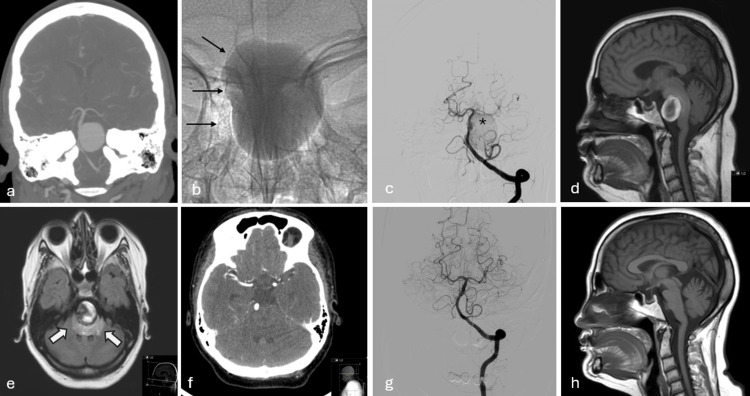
a: Coronal reformatted computed tomography angiogram shows a giant mid basilar aneurysm. b: The unsubracted image shows the Silk stent (arrows) deployed against the aneurysm neck. Note the contrast medium from the previous injection is stagnated within the aneurysm. c: Post stent vertebrobasilar angiography shows very faint opacification of the giant aneurysm (*) because of the stagnation within the aneurysm. d: The patient presented with gait disturbance and imbalance on the third month. Sagittal T1-weighted image reveals distortion of the brain stem by the thrombosed aneurysm. e: Axial fluid-attenuated inversion recovery (FLAIR) image shows severe vasogenic edema (arrows) surrounding the clotted aneurysm. f: CT angiography confirms total thrombosis of the aneurysm. g: Nine-month follow-up angiography shows no filling of the aneurysm. h: Six-year follow-up. Sagittal T1 image almost total disappearance of the aneurysm and recovery of the brain stem anatomy. A very small remnant of the sac is seen anterior to pons.
